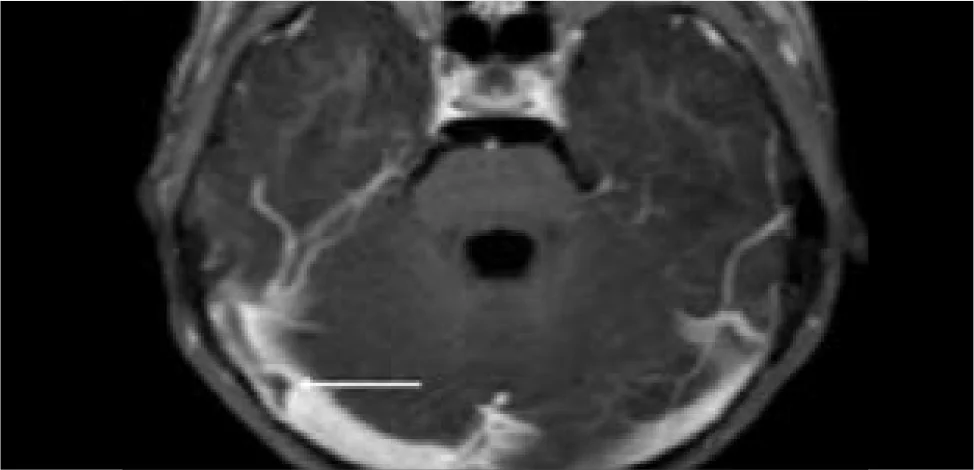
MRI of a 41-year-old woman presenting with headache. Axial T1WI
